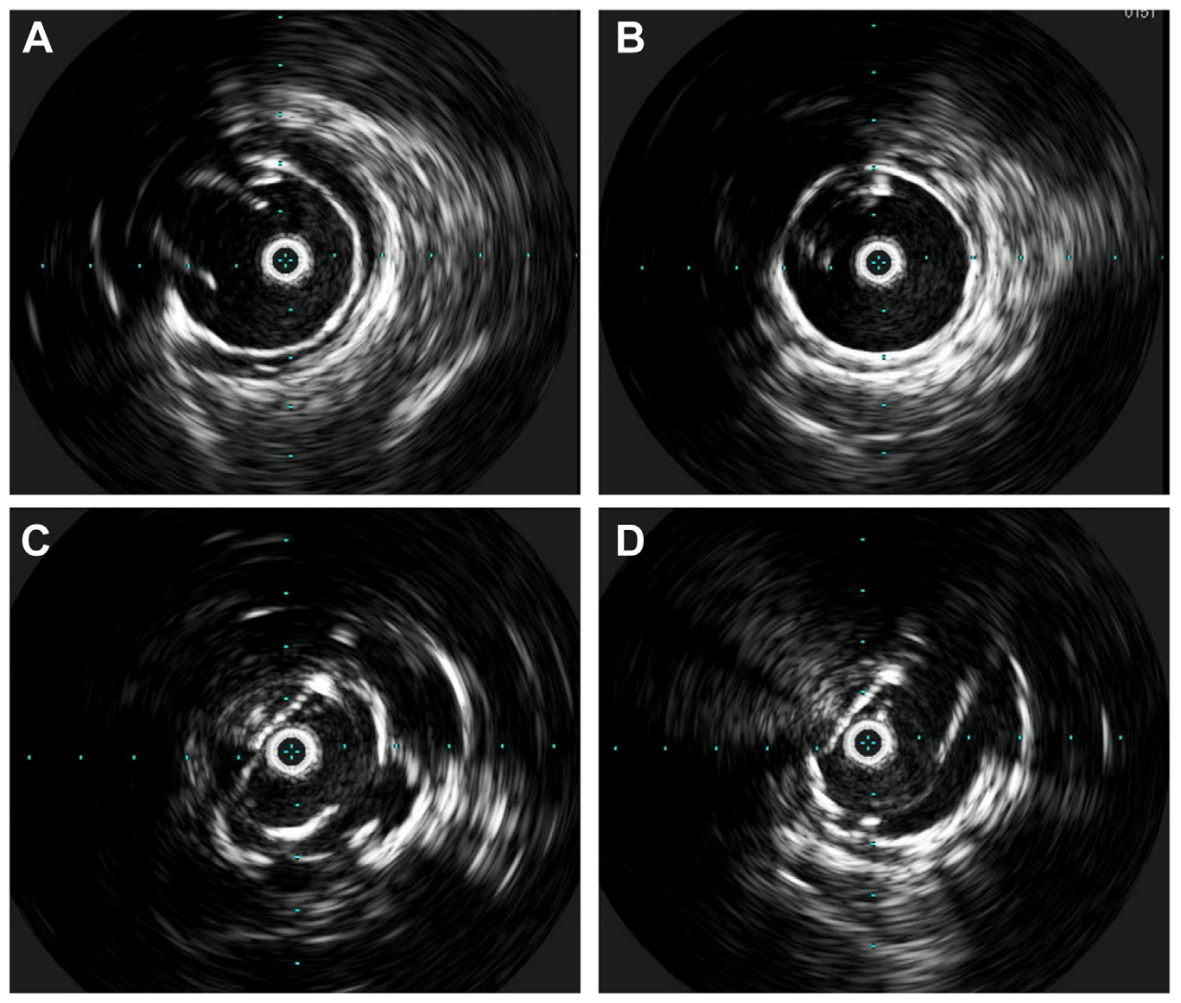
Final intravascular ultrasound (IVUS) imaging confirming visceral artery stent placement. (A) Celiac artery. (B) Superior mesenteric artery (SMA). (C) Left renal artery. (D) Right renal artery.
